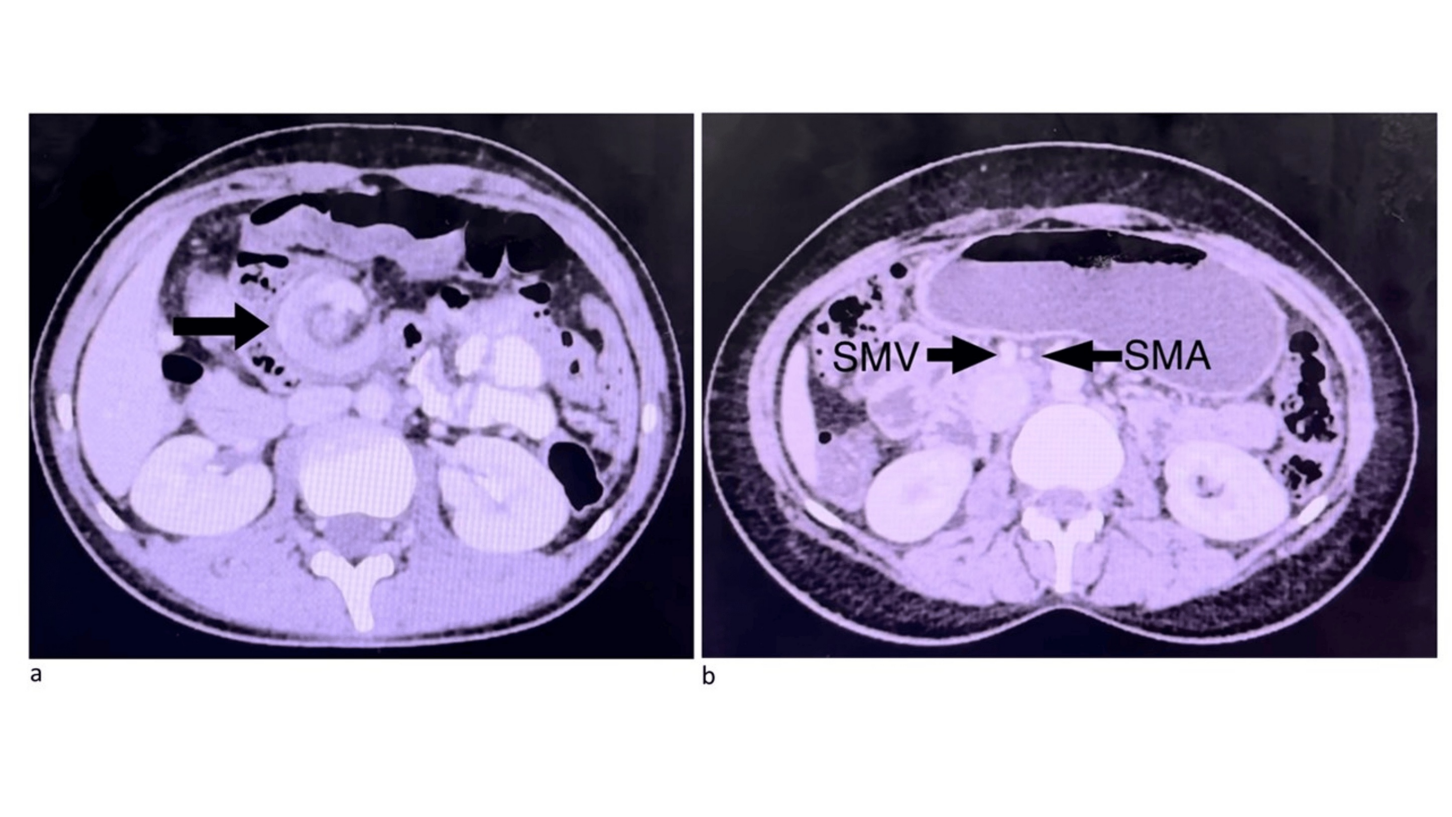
a) Axial view CECT showing classical whirlpool sign; b) Axial view CECT showing inverse relationship of SMV and SMA CECT: contrast-enhanced computed tomography, SMV: superior mesenteric vein, SMA: superior mesenteric artery
